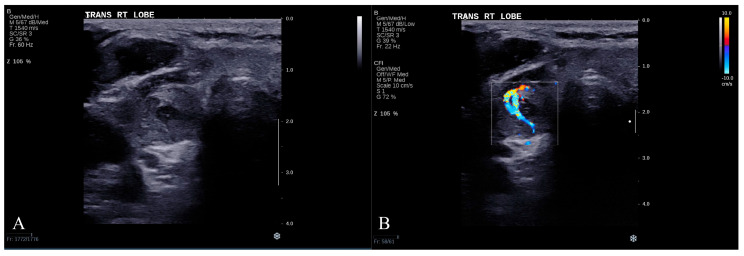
A representative misclassified case. (A) Grayscale and (B) color Doppler ultrasound images of a malignant thyroid nodule. The images are shown uncropped to preserve the full anatomical context for the reader.
